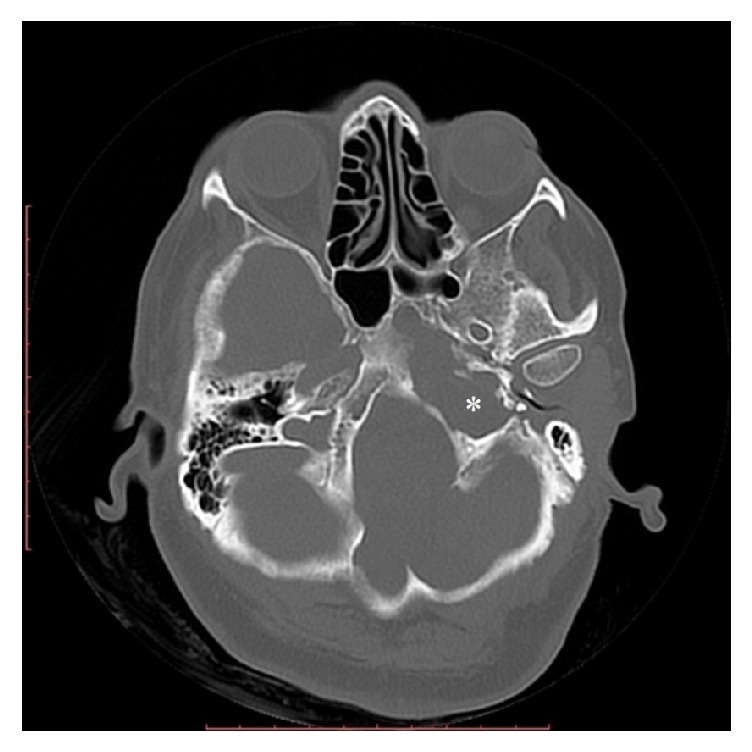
Axial CT image with the soft tissue window settings shows a low-density pouch herniated to the jugular foramen (*∗*).
